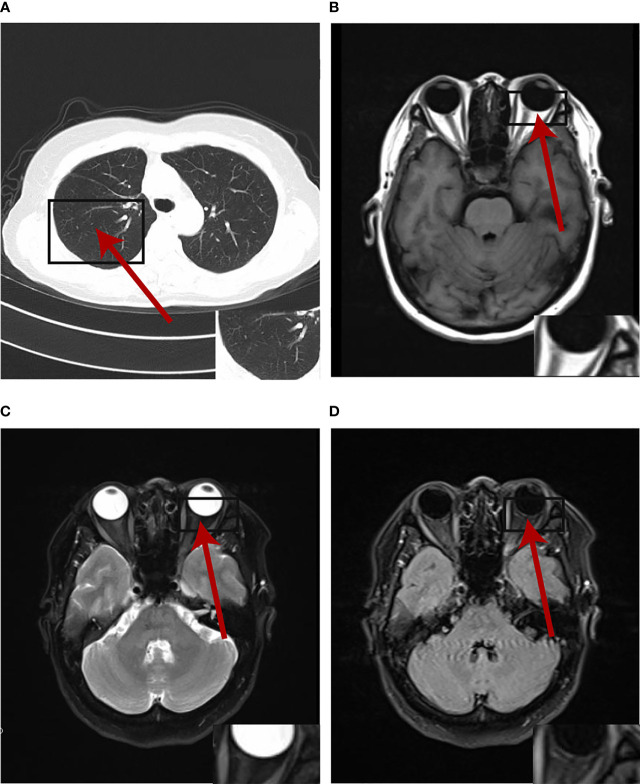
The primary lesion and oligogenous metastases were complete response by the patient's latest (August 2021) chest CT and cranial MRI at the time of writing.
